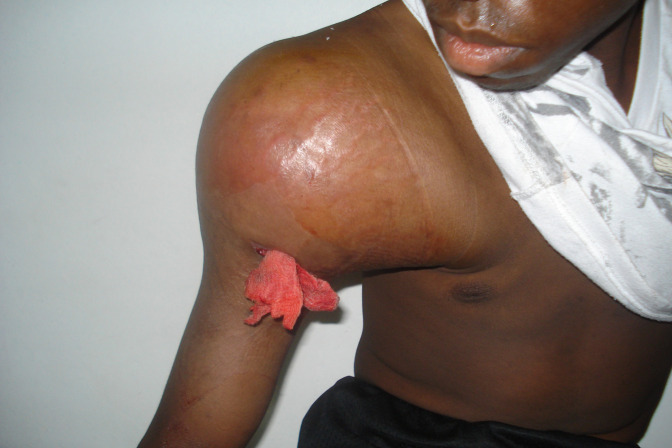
Clinical photograph of a 19-year-old man with a chronic draining wound of the right shoulder and severe pain. Radiographs of the shoulder demonstrated findings most consistent with an osteosarcoma. Given the lack of chemotherapeutic options, the patient's prognosis is poor. Owing to the risk of infection and blood loss with surgical reconstruction, palliative amputation would be an option for both resection of the tumor and pain control.
